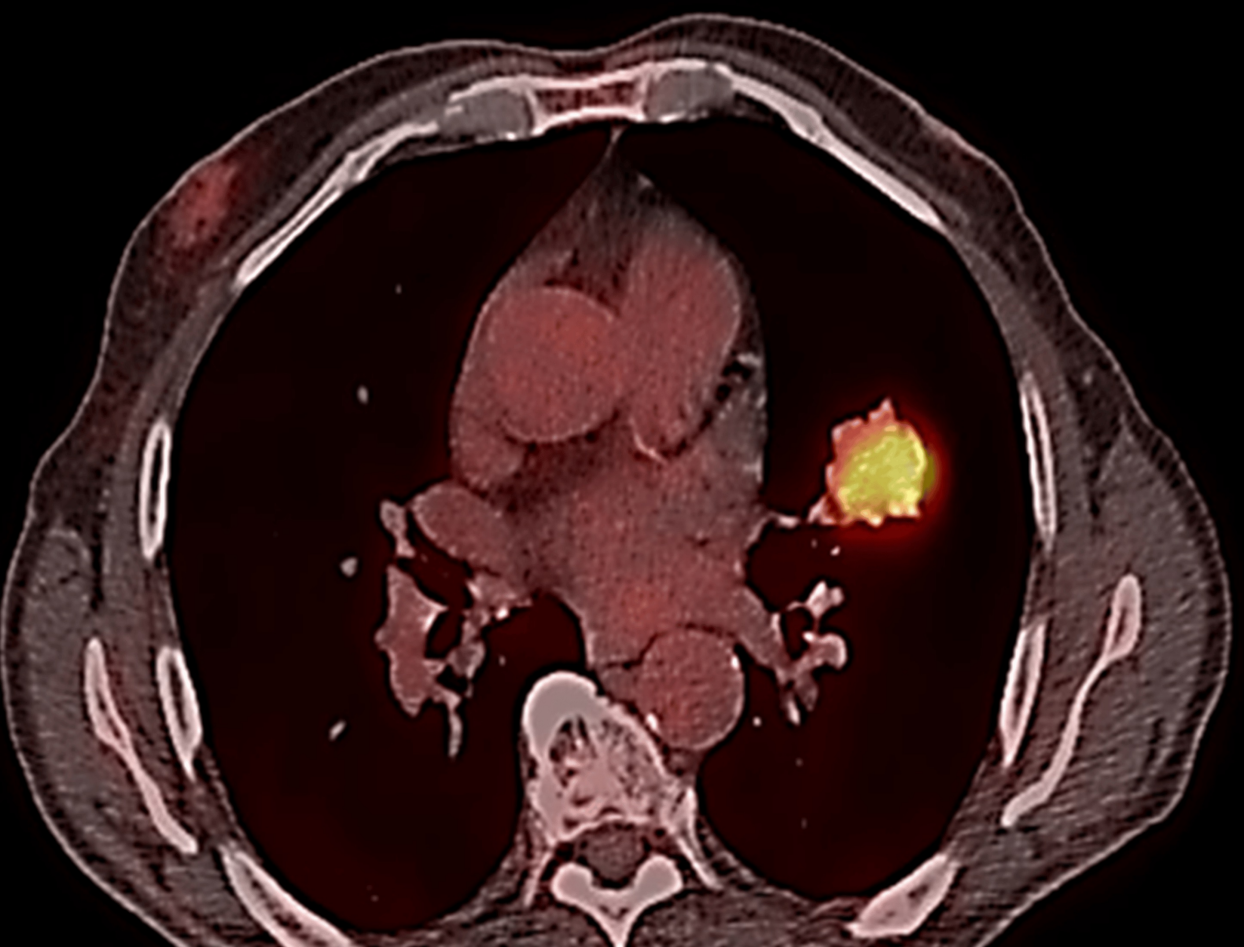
PET-CT scan showing increased uptake of FDG from the lesion (SUVmax: 8.8)
